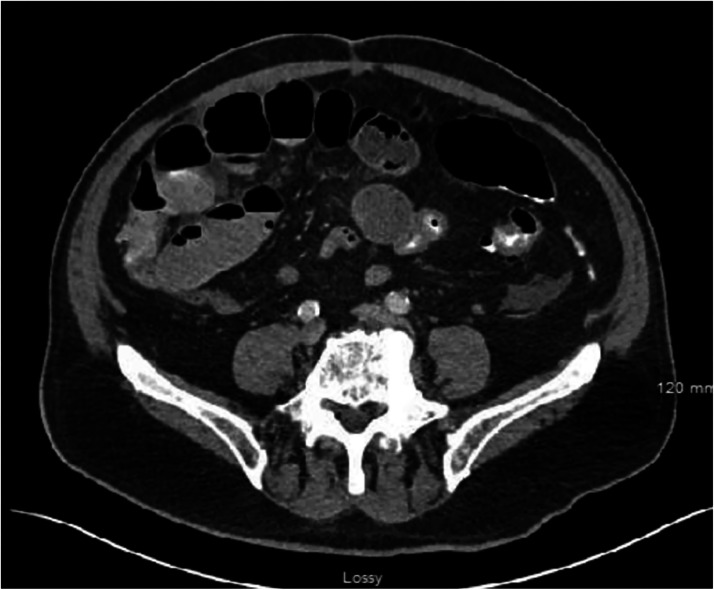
Axial abdominal CT image on admission displays findings including dilated small bowel and air-fluid levels consistent with partial bowel obstruction.
